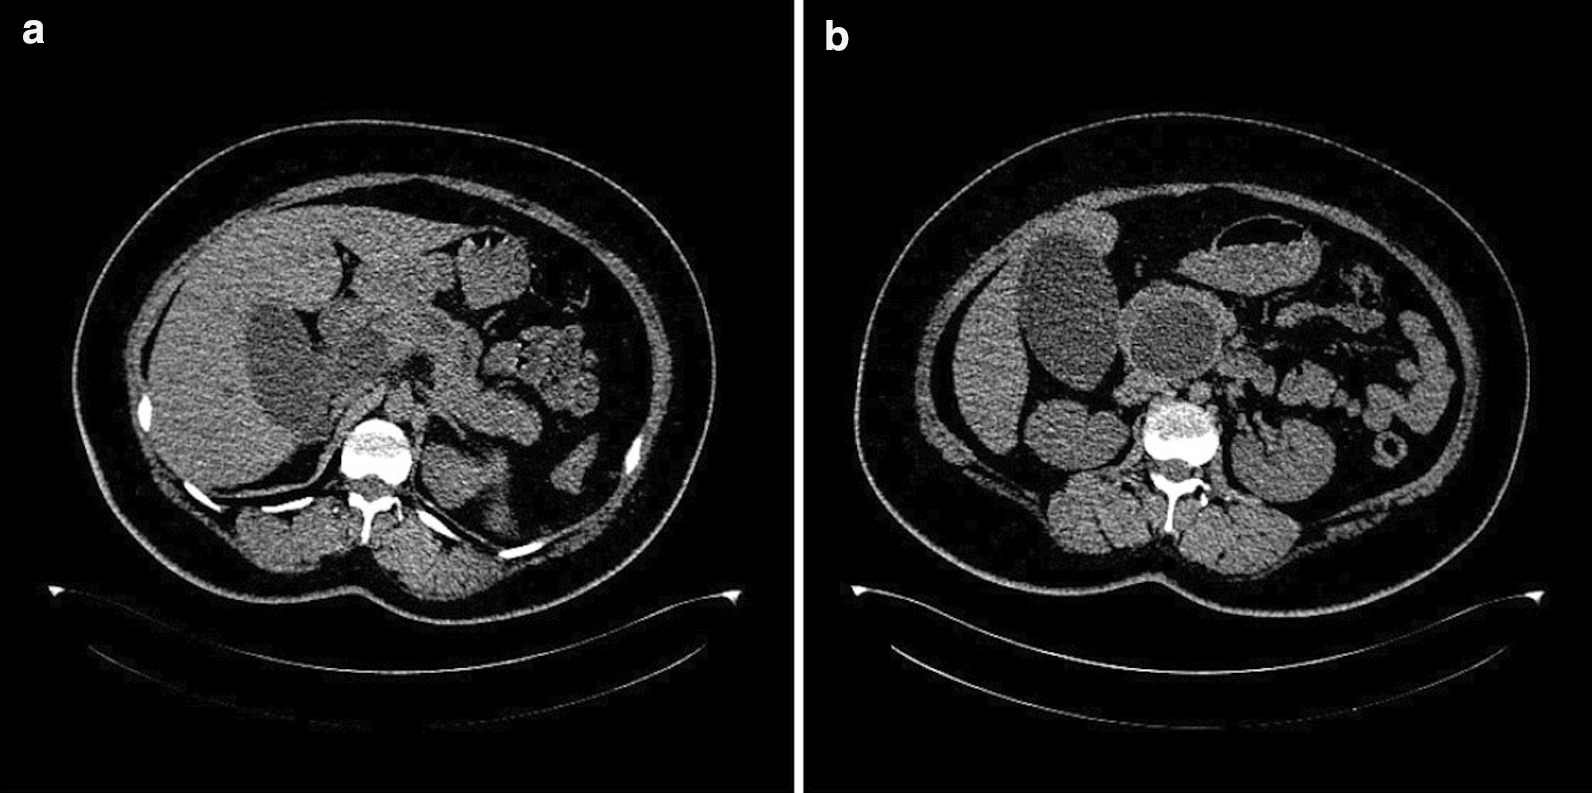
CT scans of the pancreatic hydatid cyst. a pancreatic cyst inducing bile duct and main pancreatic duct expanding; b pancreatic head hydatid cyst
